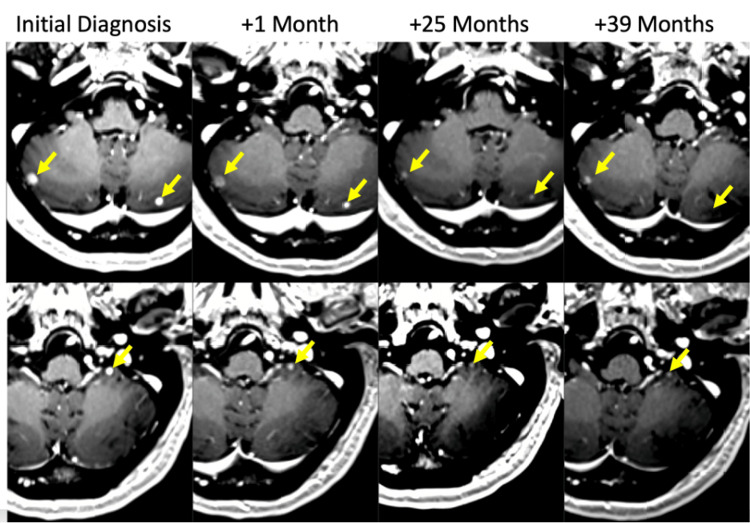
Rapid and durable response following PRRT, with deferral of brain radiotherapy or radiosurgery, of multiple cerebellar brain metastases from GEP-NET shown are representative images of three of nine total cerebellar metastases at the time of initial diagnosis and at one month following the start of PRRT; an early response is seen. These responses were durable at 25 months post-diagnosis; at this time, two additional cycles of PRRT were given due to systemic progression. The most recent MRI at 39 months displays continued stability of the treated brain metastases, with no new brain metastases. PRRT: peptide receptor radionuclide therapy; GEP-NET: gastroenteropancreatic neuroendocrine tumors
